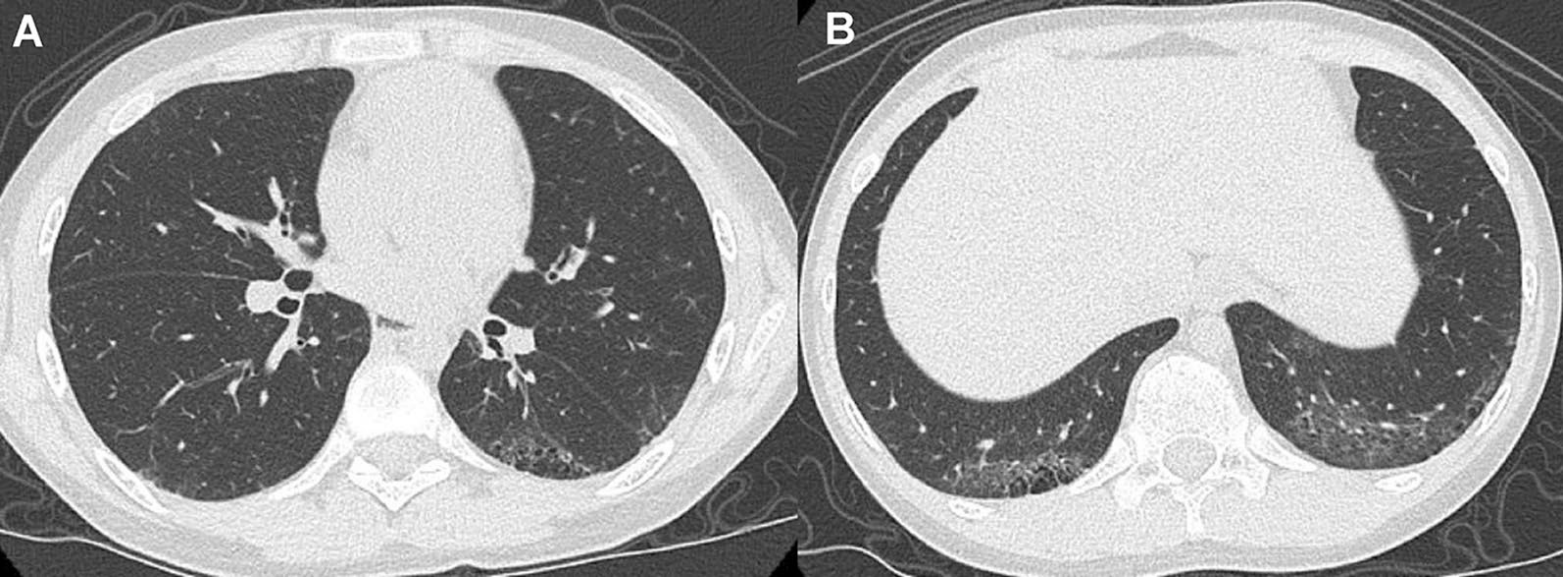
Pulmonary manifestation of SLE. A 17-year-old woman. A,B CT showed subpleural GGOs with cystic changes dependently distributed. Although the lung changes were relatively mild, the main pulmonary arteries were dilated due to lupus-associated pulmonary hypertension (not shown)
